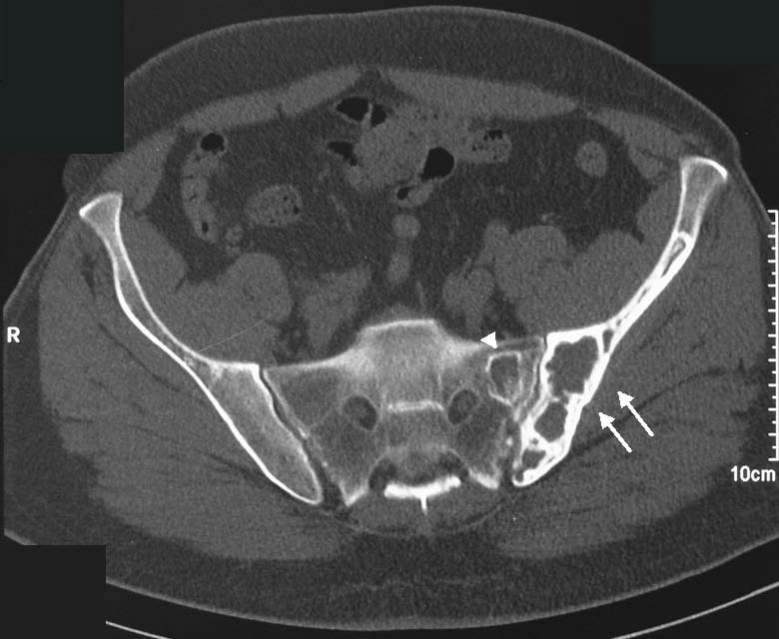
Axial CT in bone density, demonstrates a lytic expansible lesion with cortical breakthrough at the left iliac bone (arrows) and a smaller one at the left sacral wing at the level of S2 (arrowhead).
